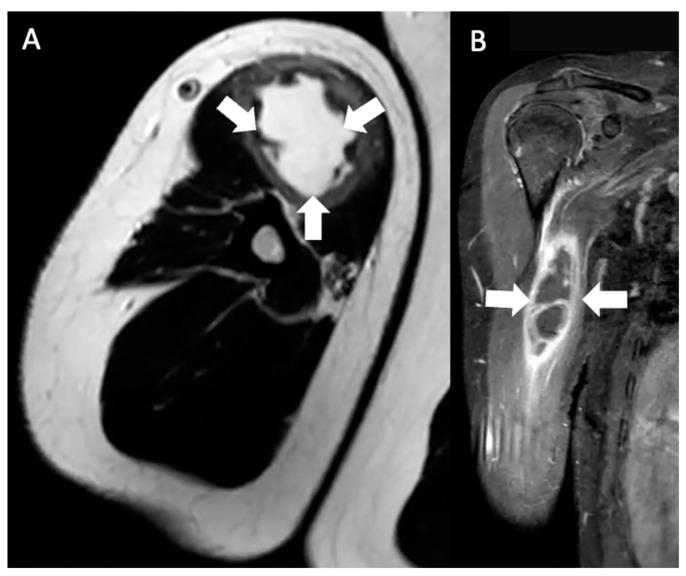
Multiplanar MRI of the right upper arm taken five days after identifying the mass and six months after receiving the booster dose. Axial T2 (A) and coronal T1W FS (B) images show an ill-defined fluid signal within the short head, right biceps muscle belly. The area involved extended from the muscle belly to the proximal humeral cortex, measuring approximately 7.4 × 3.7 × 2.6 cm (arrows).
